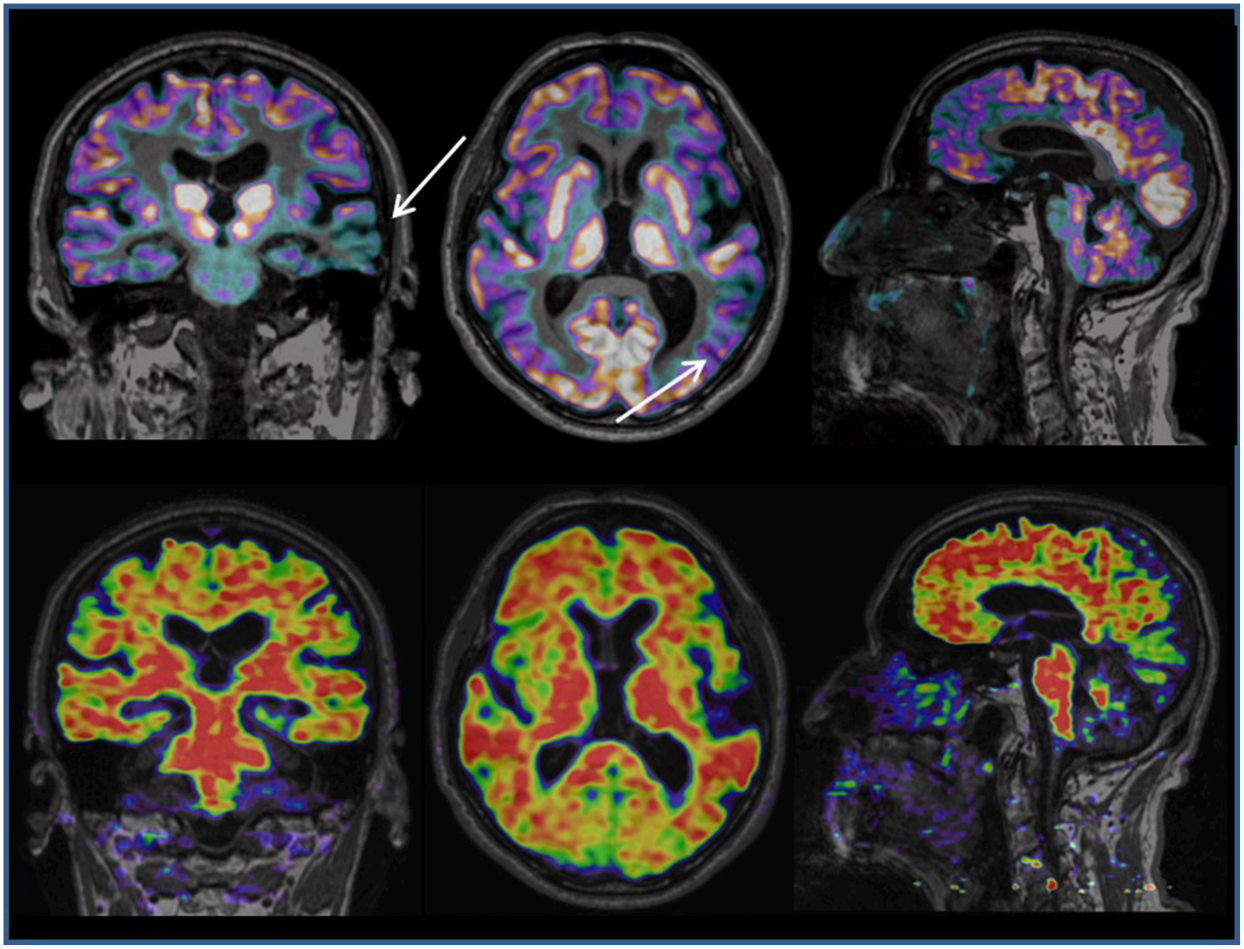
Hybrid PET/MRI imaging (Biograph mMR, Siemens). The picture above shows MRI 3D T1-weighted and 18FDG PET coronal, axial and sagittal slices in a patient affected by progressive speech disorder. The combined structural and metabolic image shows focal atrophy and reduced glucose metabolism in the left temporal lobe (white arrows), suggesting a diagnosis of Primary Progressive Aphasia, a rare form of dementia. The picture below shows MRI 3D T1-weighted and a 18F-flumetamole PET coronal, axial and sagittal slices in the same patient. The combined structural and molecular (Aß amyloid accumulation) image shows a diffuse increase in Aß amyloid deposits in the cortex, supporting the diagnosis of brain neurodegenerative disease.
